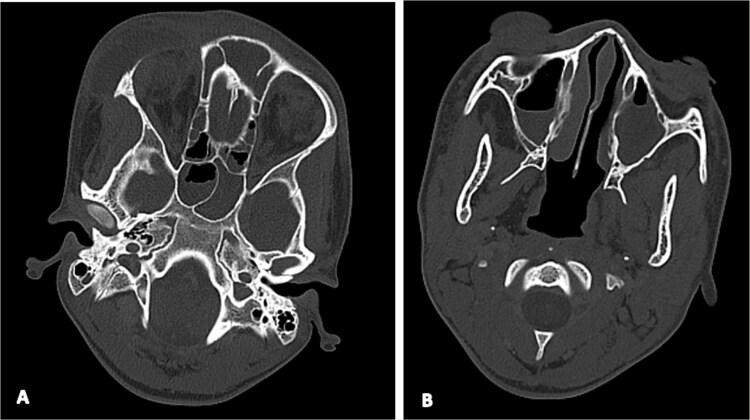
Axial brain CT (bone window) showing ethmoido-frontal sinusitis (A) and bilateral maxillary sinusitis (B).
